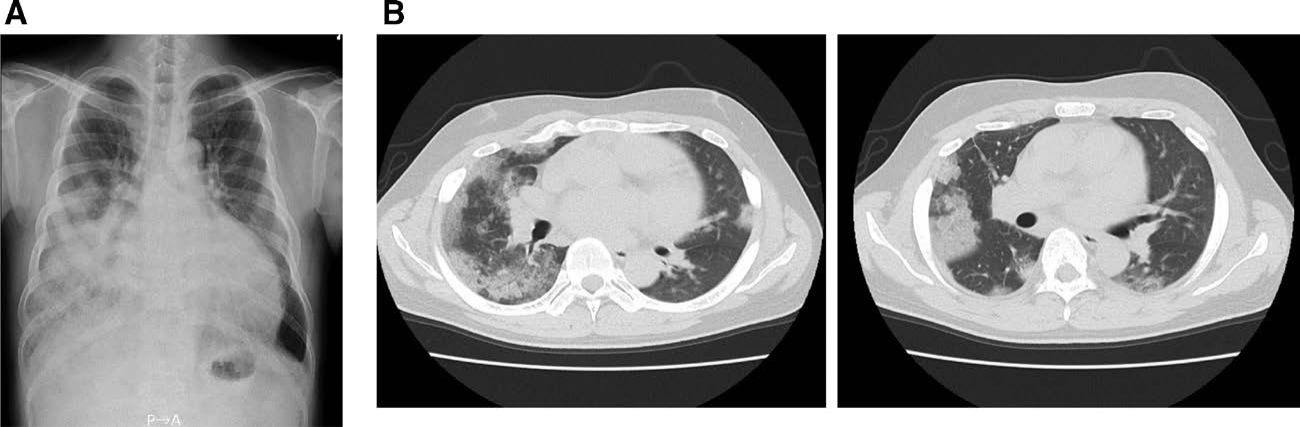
(A) Chest X-ray showing right lung field infiltration. (B) Chest computed tomography showing diffuse bilateral infiltration shadows.
